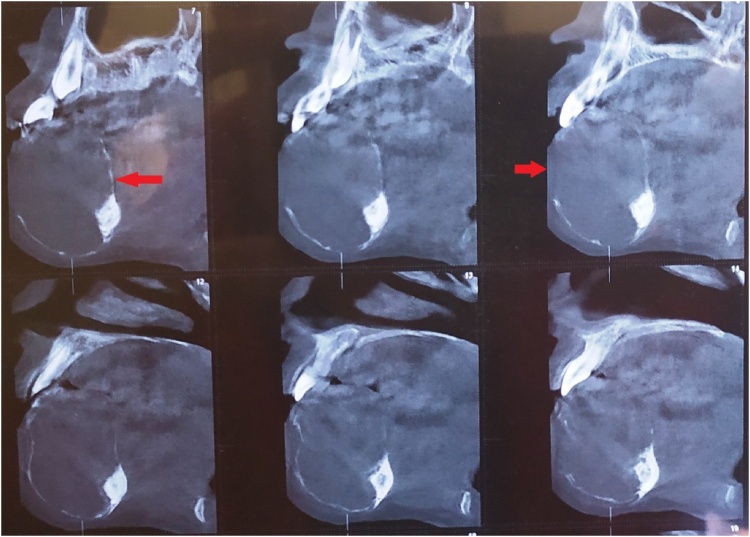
CT scan (sagittal cut) showing the mandibular mass (arrow).
